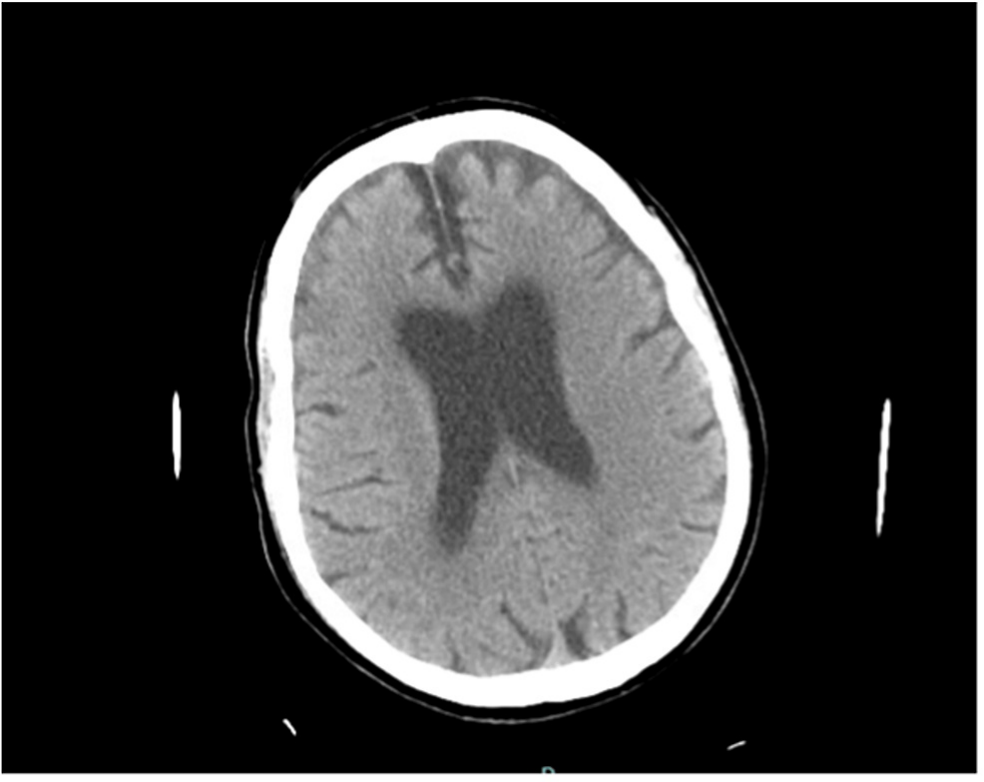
Computed tomography of the head on admission
